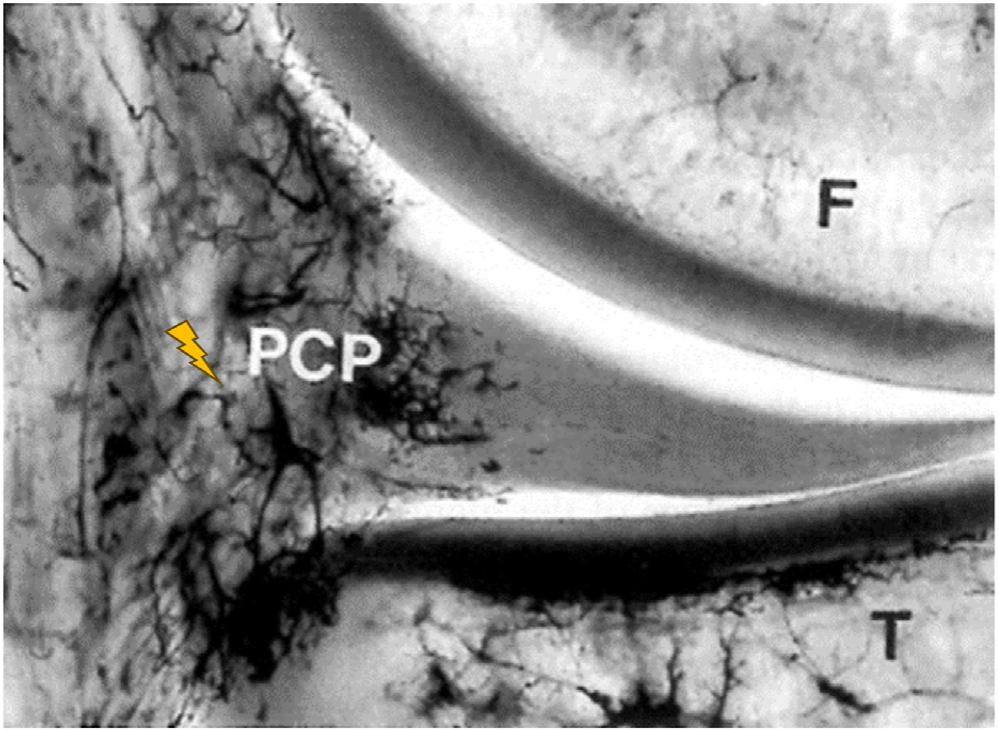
Vascularization of the meniscus (coronal view). The ultrasound-guided injection is targeted on the trigger area (yellow flash), which is located near the perimeniscal capillary plexus. Reprinted from Arnoczky and Warren^15^ by permission of Sage Publications. (F, femur; PCP, perimeniscal capillary plexus; T, tibia.)
